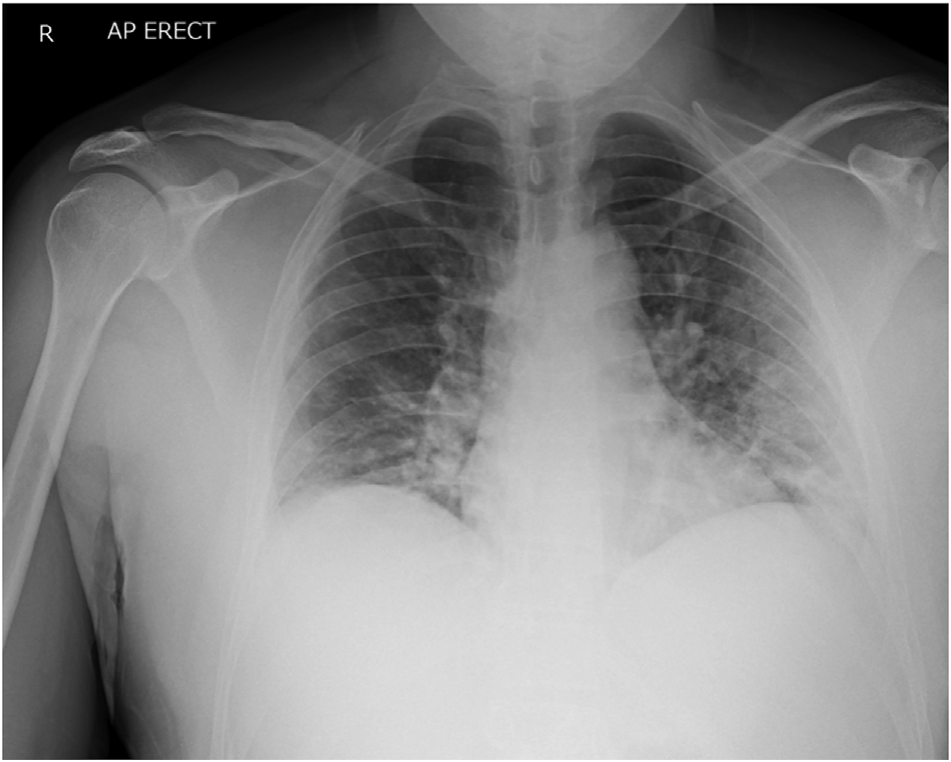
Chest radiograph from day of admission of 38 year-old male presenting with 7 day history of cough and fever. Radiograph shows bilateral infiltrates.
